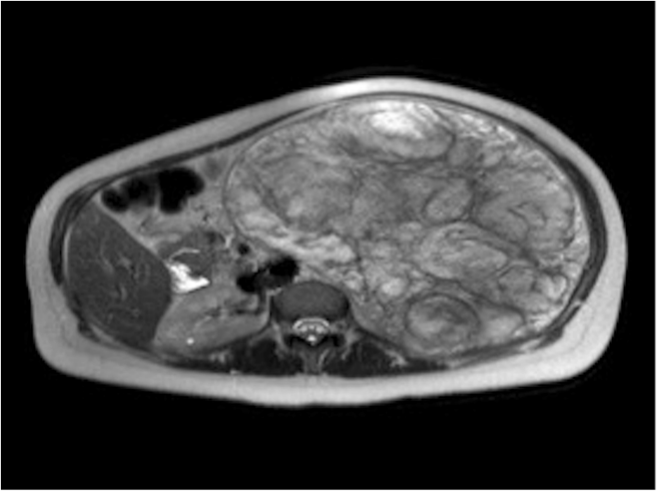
Axial T2-weighted magnetic resonance imaging of splenic hamartoma.
